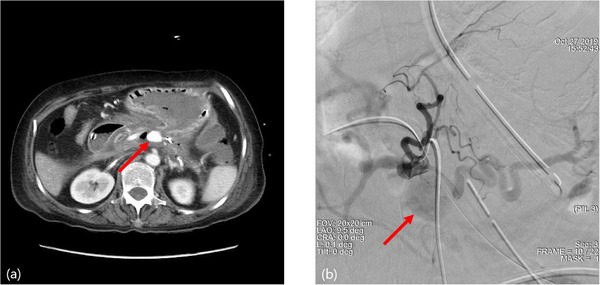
Adverse events of splenic pseudoaneurysm rupture after lumen‐apposing metal stents (LAMS) placement. (a) Eighty days after LAMS placement, the patient experienced massive hematemesis and fell into shock. Contrast‐enhanced computed tomography revealed that a splenic pseudoaneurysm (red arrow) had developed after LAMS placement. (b) Interventional radiology confirmed a splenic pseudoaneurysm (red arrow). The patient underwent hemostasis by embolization with interventional radiology.
